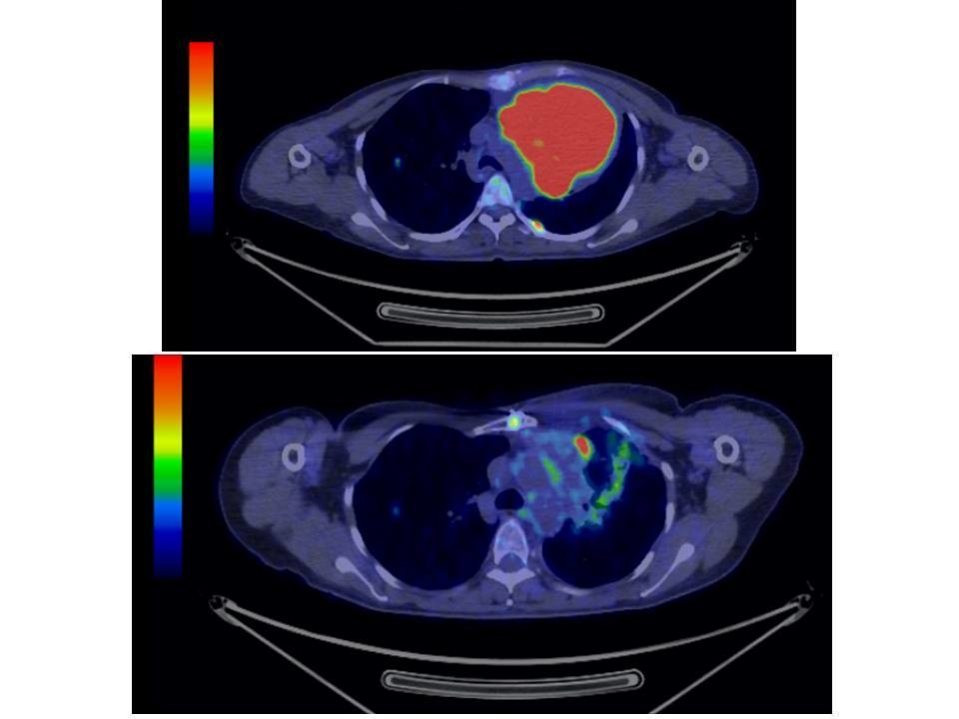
FDG-PET of Case 4 Before SBRT (top) and after SBRT (bottom).
